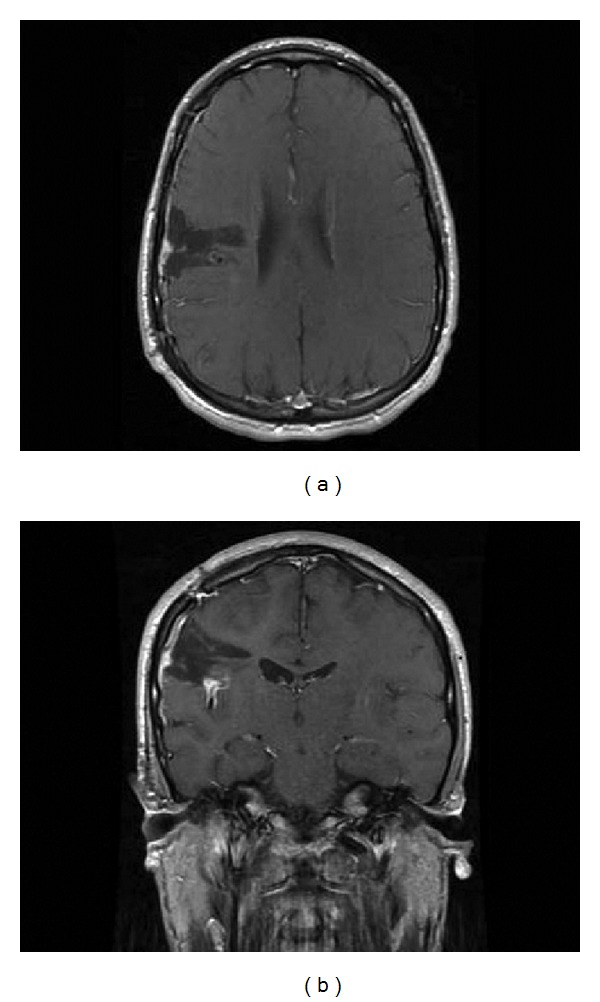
T1-weighted axial (a) and coronal (b) MRI at the 1-year postoperative visit show encephalomalacia, no increase in size of residual lesion, and persistent enhancement in sylvian cistern.
